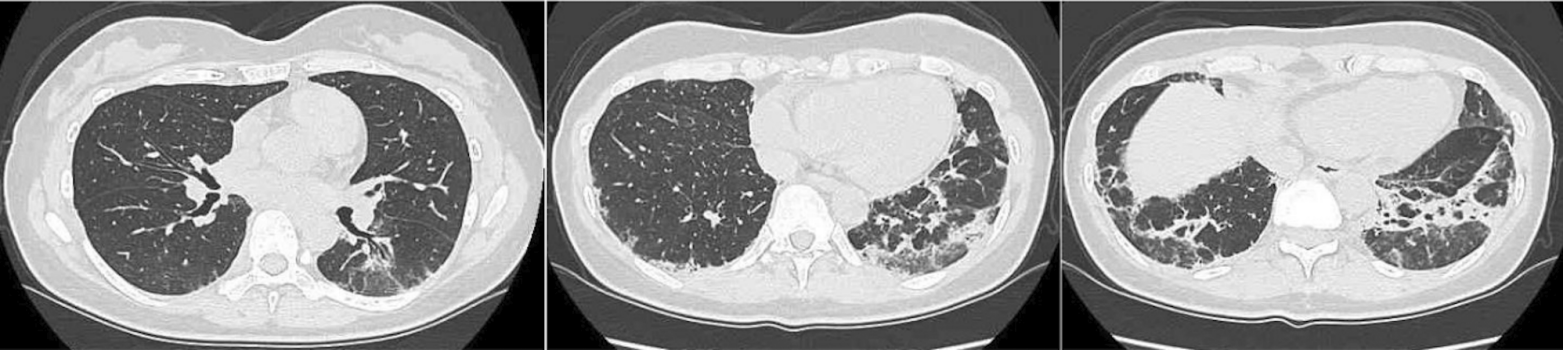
Chest computed tomography during patient's first visit.
